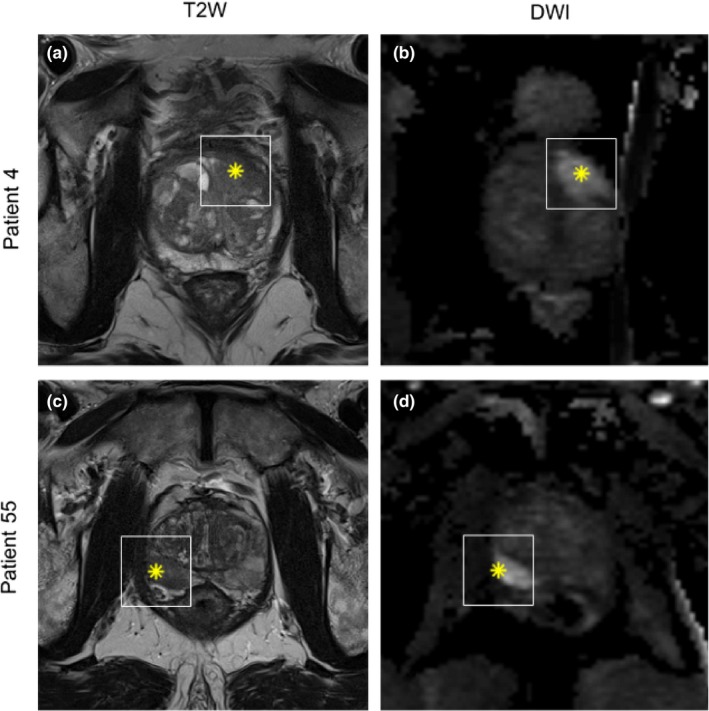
Example of region of interest (white square) around lesion from patient 4 (a and b) with a lesion located in the anterior fibromuscular stroma and patient 55 (c and d) with a lesion in the peripheral zone. Left column is T2W and right column DWI sequence. Asterix inside region of interest denotes the point from where the prostate biopsy was obtained.
